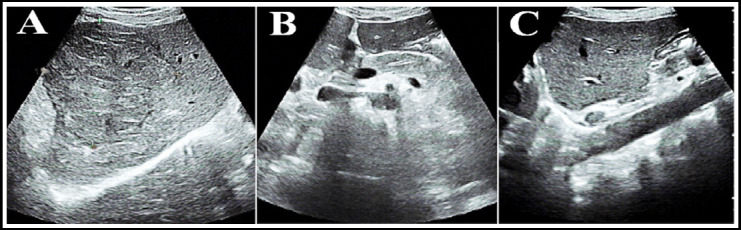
Ultrasound images. *Note:* A was an ultrasound image of a female patient (age 68 years old ); B was that of a male patient (age 54 years old); C was that of a male patient (age 57 years old).
